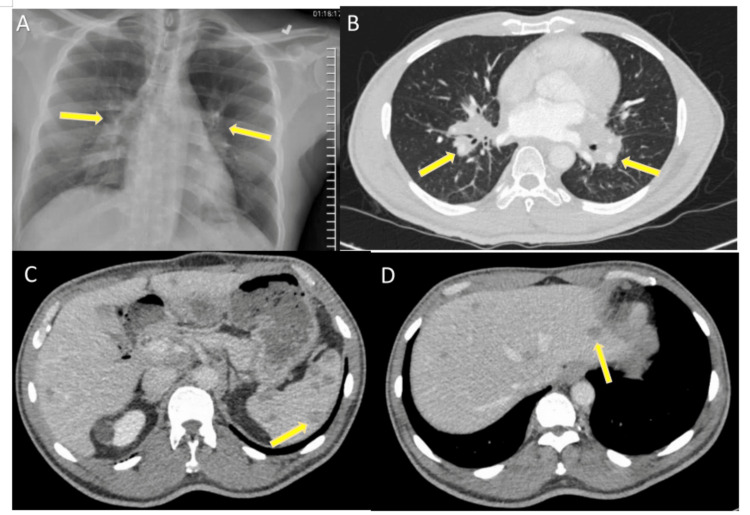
(A) Chest X-ray showing bilateral hilar fullness. (B) Chest CT with contrast showing confluent hilar/mediastinal soft tissue mass. (C,D) Abdominal CT with contrast showing innumerable hypodense lesions throughout the liver and spleen. Imaging findings are marked with yellow arrows. CT: computed tomography
